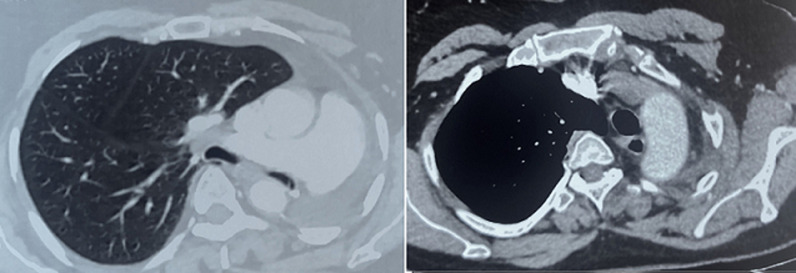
monitoring by thoraco-abdominal-pelvic CT scan after 1 year of pneumectomy
